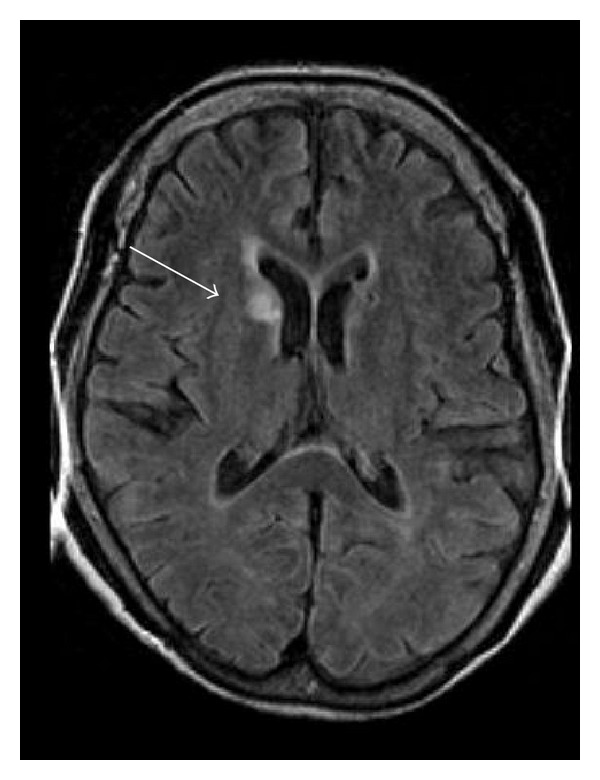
Magnetic resonance imaging of the brain shows a focal lesion in the right caudate head, which shows hyperintensity on fluid-attenuated inversion recovery imaging.
